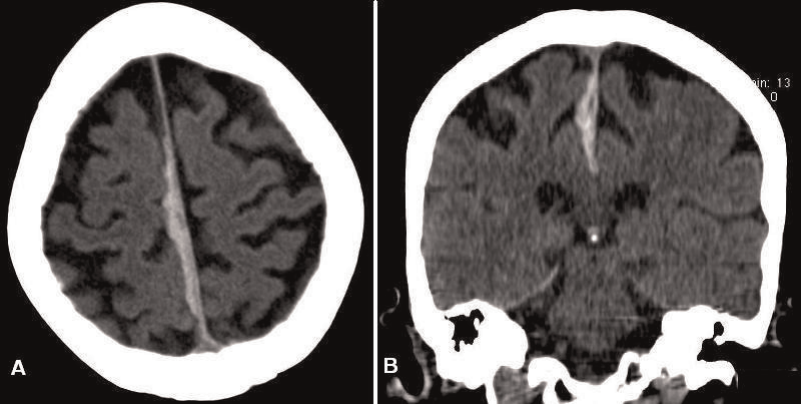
(A,B) Computed tomography of the brain on admission shows a 5 mm thick linear hyperdense lesion along the right side of the interhemispheric fissure.
